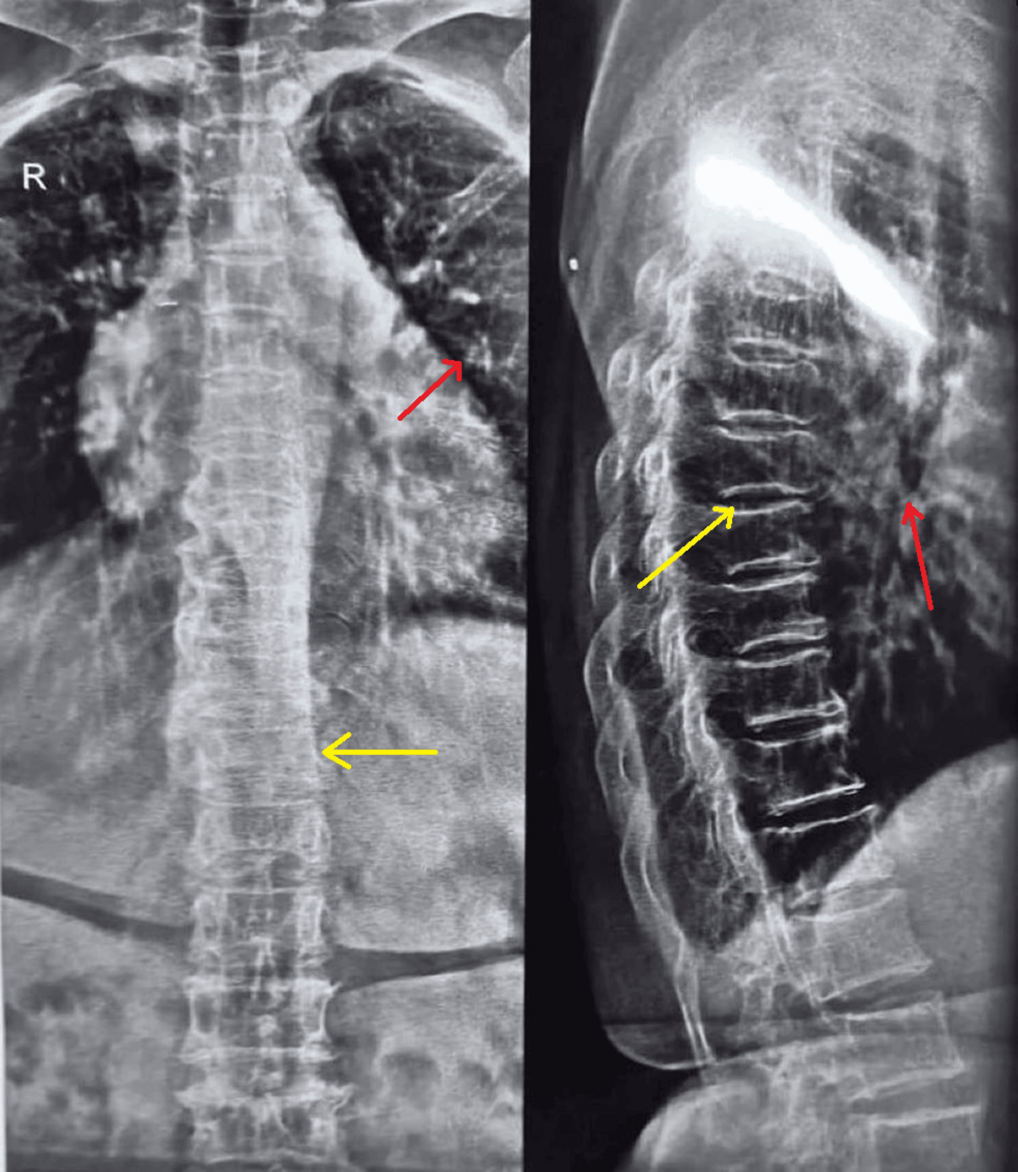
X-ray showing kyphoscoliotic changes in the spine and chronic obstructive pulmonary disease (COPD) changes in the lungs Red arrows showing chronic obstructive pulmonary disease lung; Yellow arrows showing kyphoscoliotic spine.
